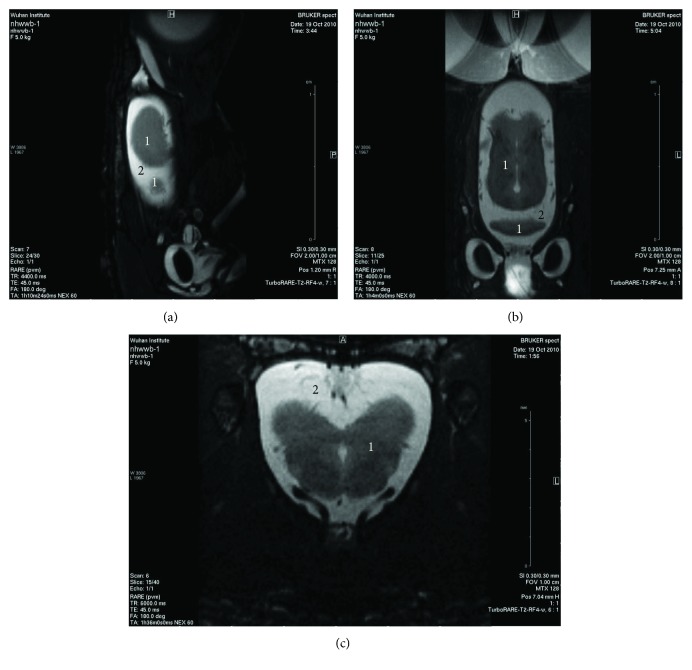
The MRI scanning of a gecko head in the sagittal plane (a), horizontal plane (b), and coronal plane (c). The dark area (marked by “1”) indicates the brain parenchyma while the bright area (marked by “2”) the CSF.
